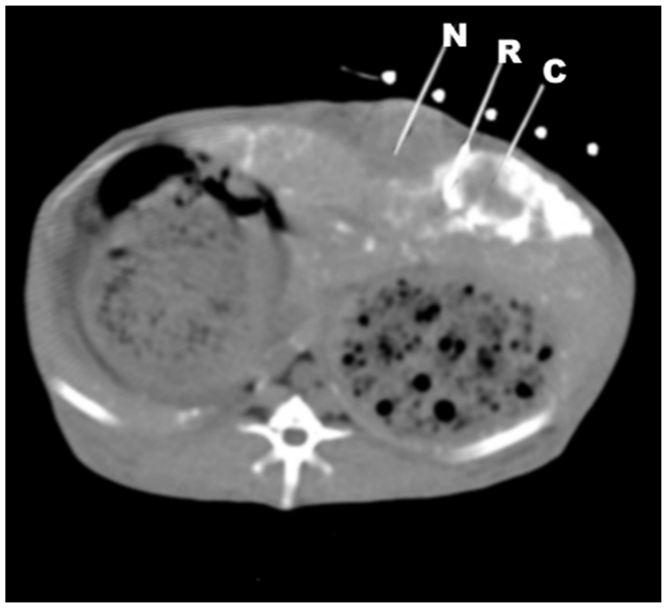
CT-guided percutaneous puncture to monitor temperature
